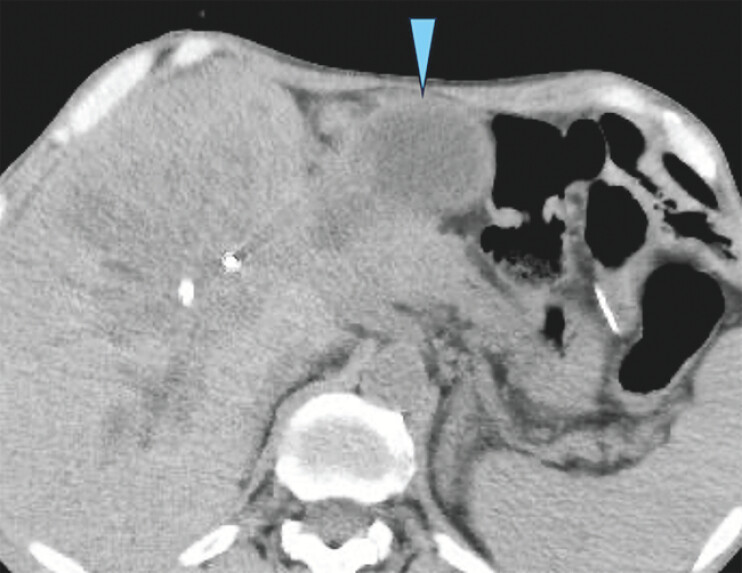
Computed tomography showing afferent loop obstruction (arrowhead).
